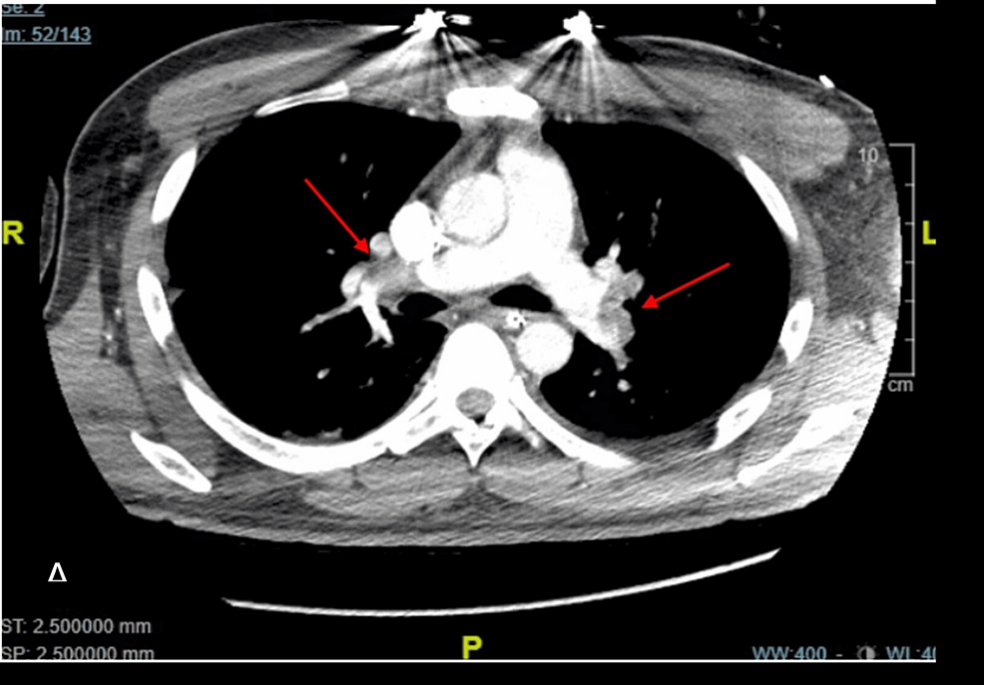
CT pulmonary angiography (axial view) This axial section of a CT angiogram clearly shows the presence of a pulmonary embolism, with arrows indicating the clot obstructing the pulmonary artery bilaterally.
